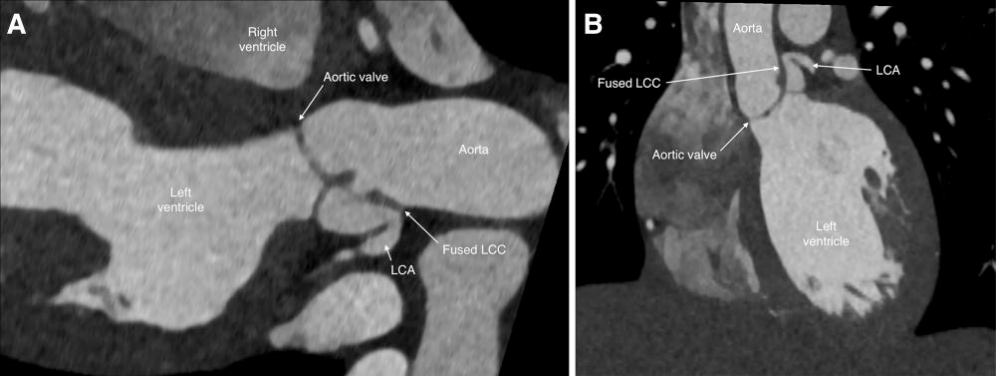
Long axis (*A*) and coronal (*B*) reconstructions from computed tomography angiogram demonstrating the fused left coronary cusp (LCC) and pathway to the left coronary artery (LCA).
